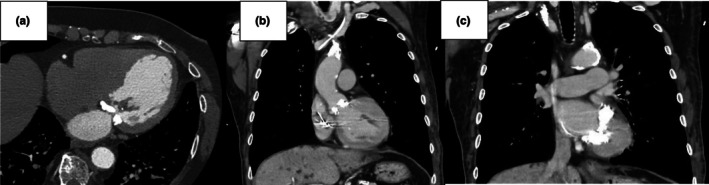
Cardiac CT scan. Cardiac CT showing calcific deposits on the mitral valve in high‐definition images, with involvement of both the anterior and posterior emiannuli (first panel); other cardiac CT images revealing a severe case of MAC, with huge calcifications of the mitral annulus and leaflets but also diffuse calcifications of the aortic valve and innominate artery, with some plaques in the ascending aorta (last two panels).
